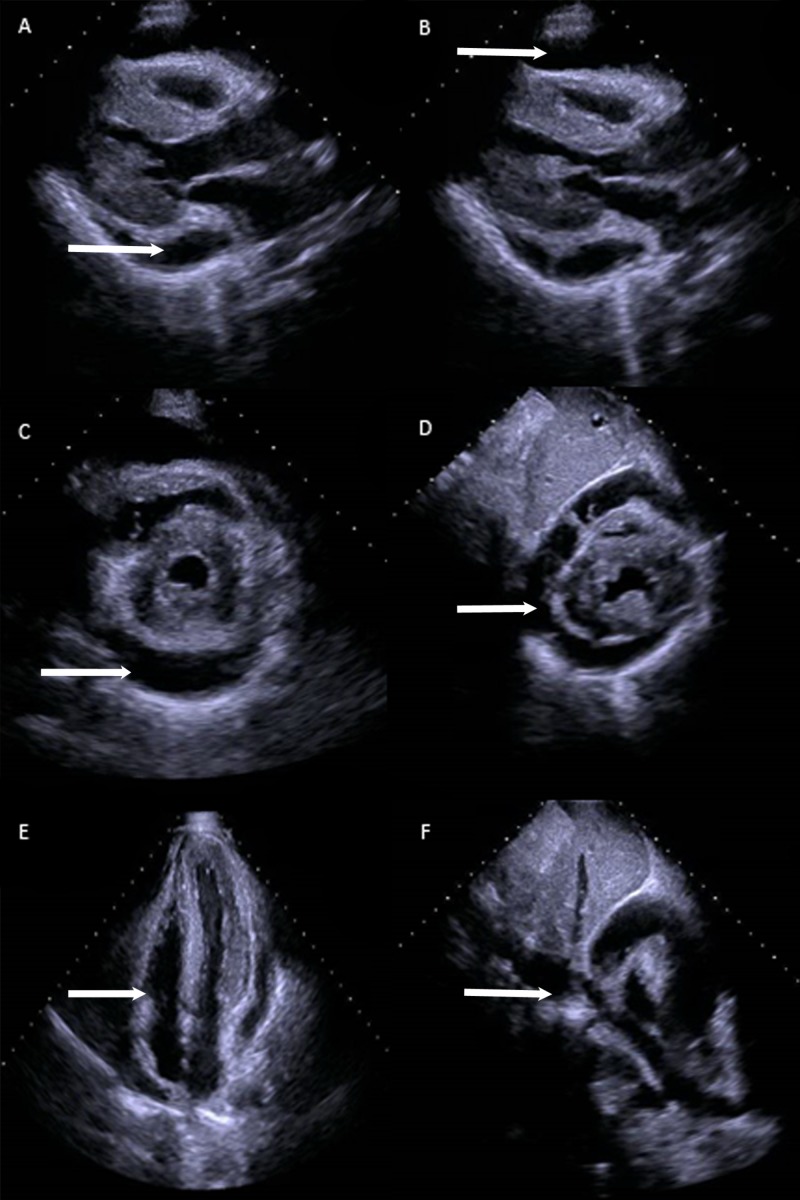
Echocardiogram. Echocardiographic findings demonstrating pericardial effusion in parasternal long view (A, B) and short axis view (C,D), flattening of interventricular septum and right ventricular collapse (E), dilated Inferior Vena Cava (F) as indicated by arrows.
